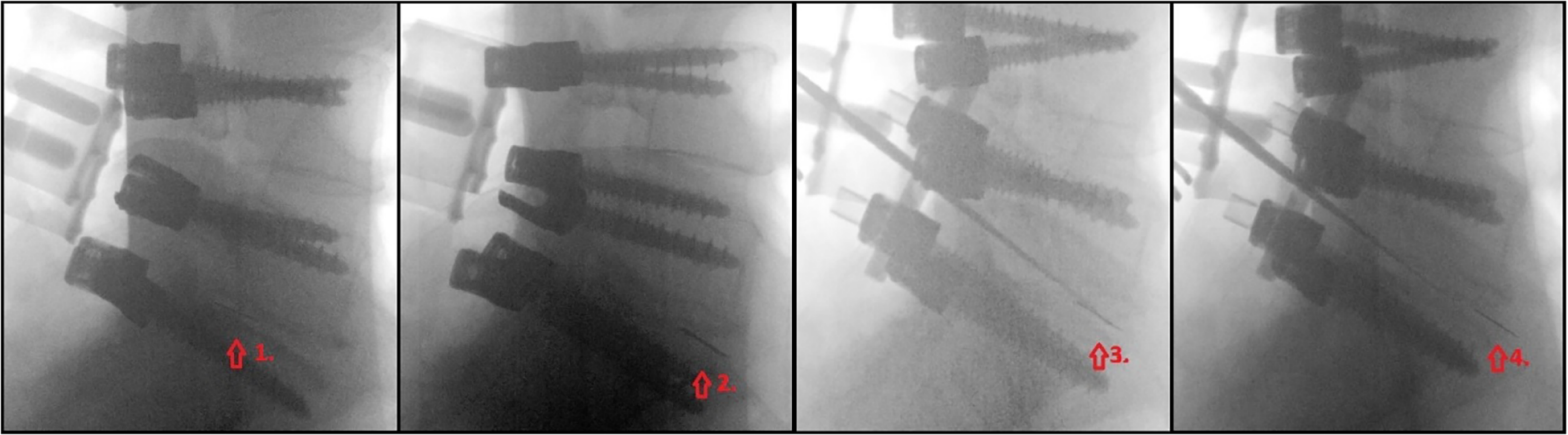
Sequential x-ray images of the anterior blade pushing technique.
